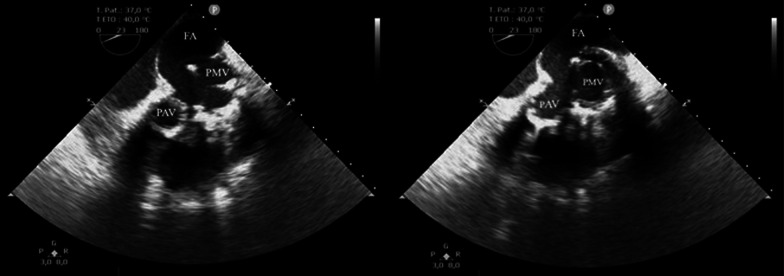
Transgastric transoesophageal echocardiography views; the false aneurysm is developed behind the prosthetic mitral valve and the left atrium. FA: false aneurysm, PAV: prosthetic aortic valve, PMV: prosthetic mitral valve
